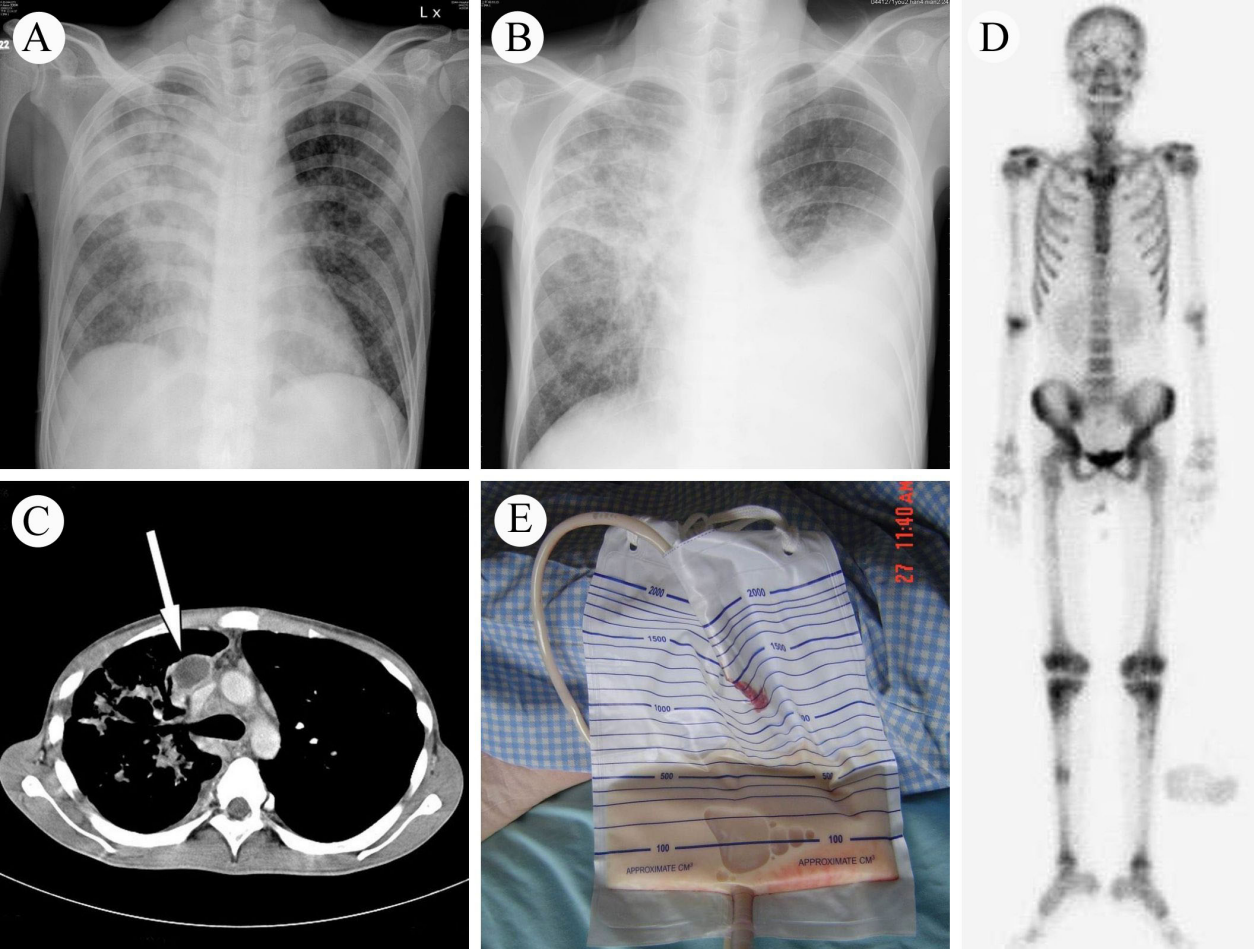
The immune reconstitution inflammatory syndrome in a patient with human immunodeficiency virus and *Mycobacterium tuberculosis *coinfection. (A) Chest X-ray on admission. (B) Chest X-ray 3 weeks after initiation of antiretroviral therapy. (C) Computed tomography of chest showed a large lymphadenopathy in mediastinum (arrow). (D) Tc-99 m methylene diphosphonate whole body bone scan showed multiple increased radioactive lesions in the axial and appendicular skeleton. (E) The drained chylous left pleural effusion.
